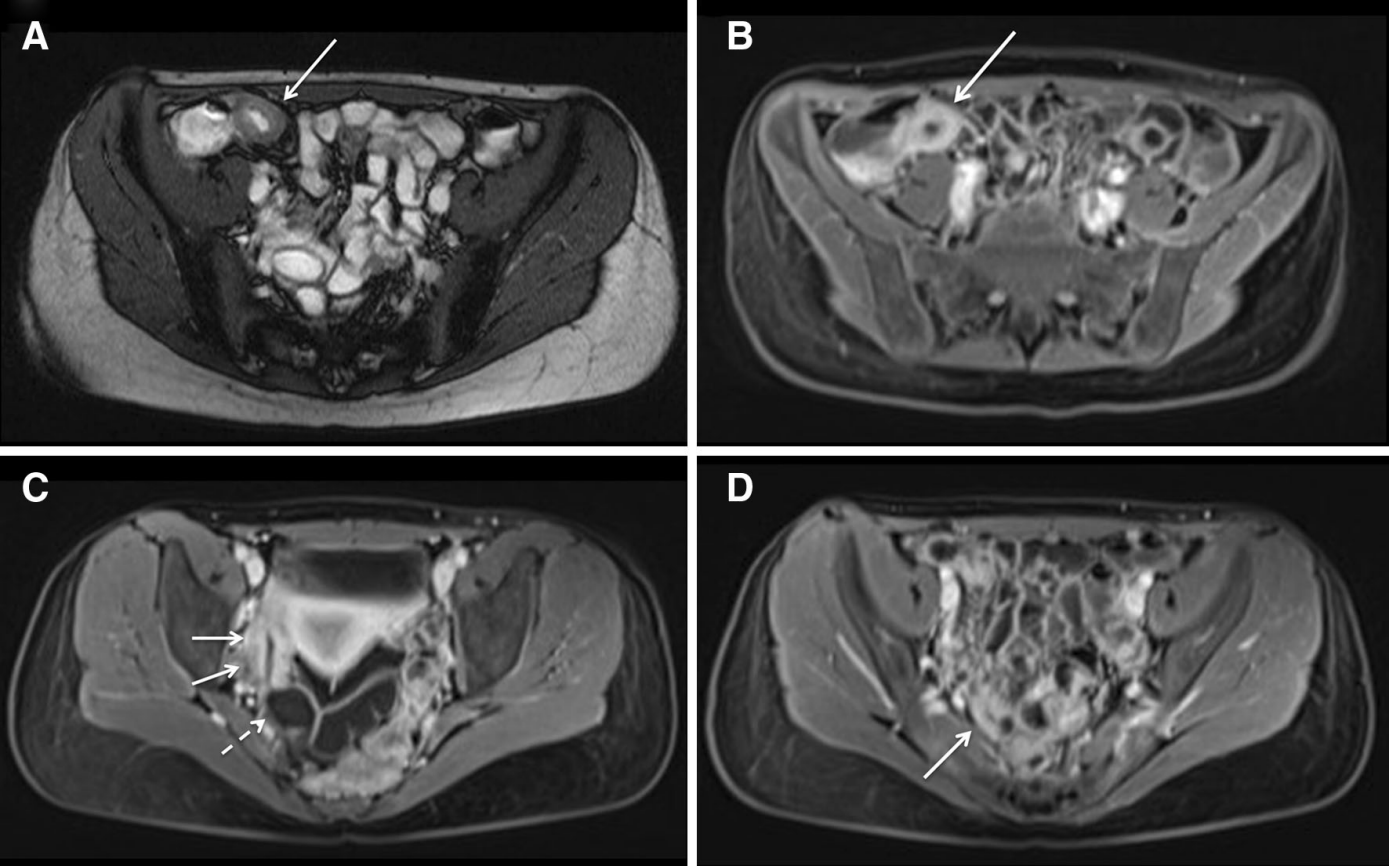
Magnetic resonance images: a Axial T2 trufi of the pelvis and b axial T1 post contrast at the same level, revealing layered bowel wall thickening with post-contrast enhancement of the terminal ileum (*arrow*). c Axial T1 post contrast shows a skip lesion with a length of 6 cm, proximal in the ileum, with bowel wall thickening and post-contrast enhancement (*arrow*); also, some prestenotic dilatation of the bowel is seen (*dashed arrow*). d Axial T1 post contrast shows a second skip lesion, with a length of 2 cm, more proximal in the ileum (*arrow*)
